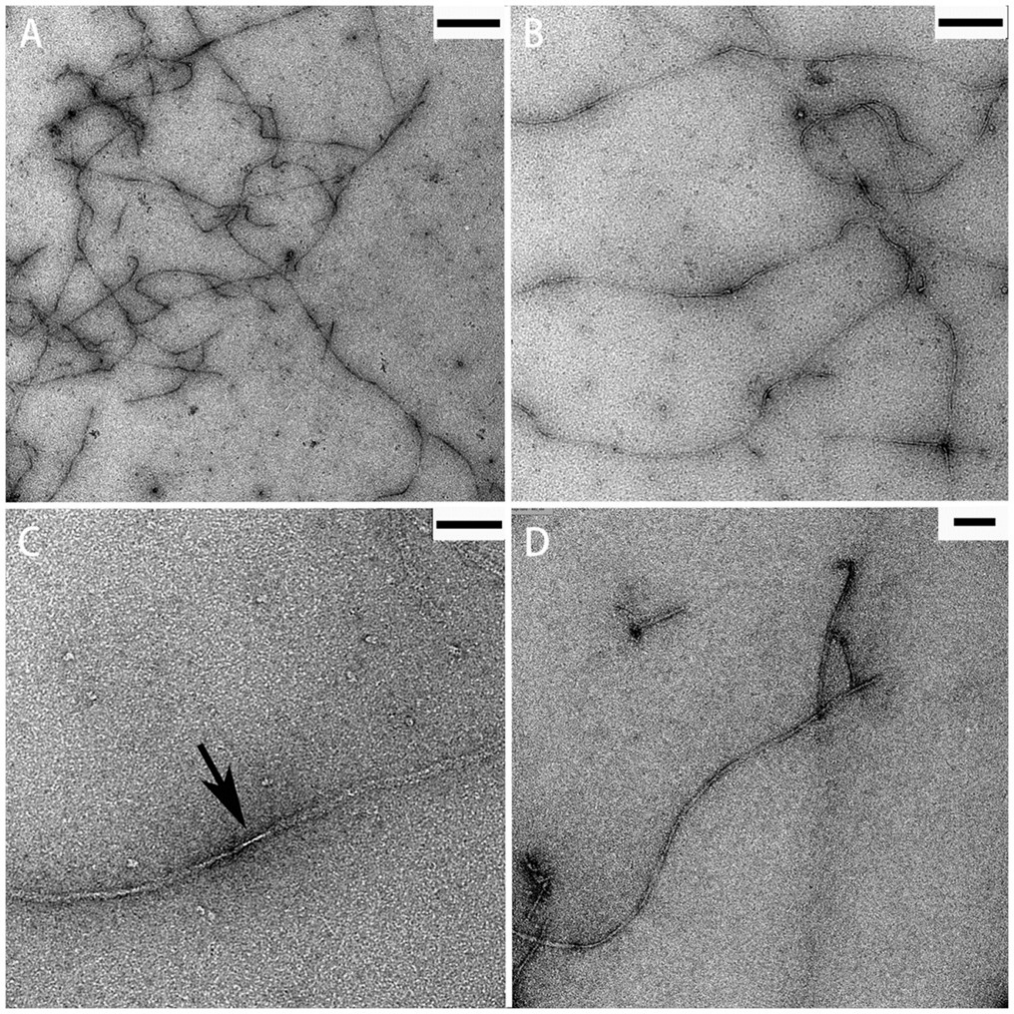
TEM images of self-assembled fibrils. A-C) RiAFP-m9 fibrils formed after incubation at 37 °C. The arrow indicates the twist of a single fibril. D) SBAFP-CT fibrils. Scale bar: A) 500 nm; B) 200 nm (resolution 3.5–3.8 nm); C) 60 nm; D) 100 nm.
